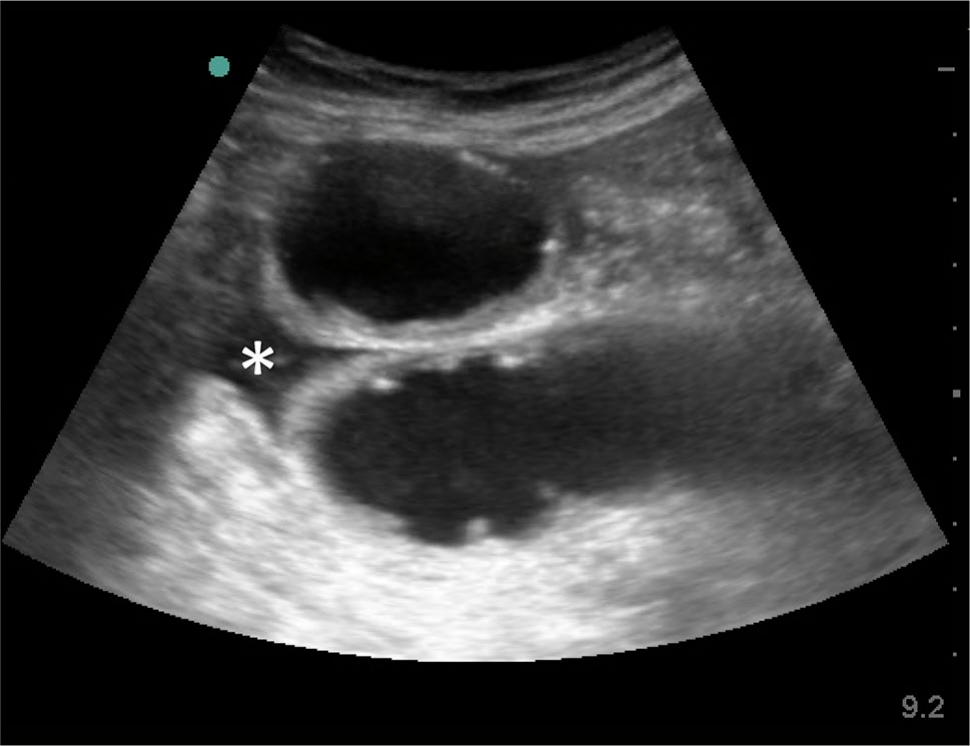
Point of care ultrasound for acute abdomen showed localized free fluid * with dilated small bowel loop in right lower quadrant with absent peristalsis
